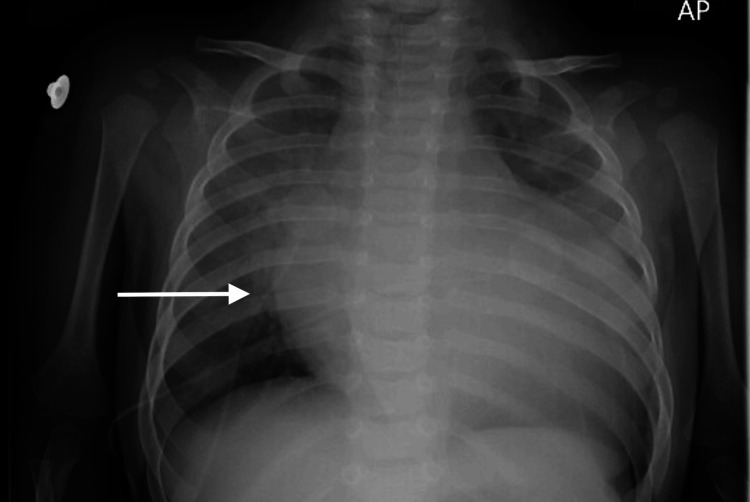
Chest X-ray. Arrow indicating cardiomegaly on the chest X-ray of a patient presenting with signs and symptoms concerning for myocarditis and cardiogenic shock. Courtesy of the authors
